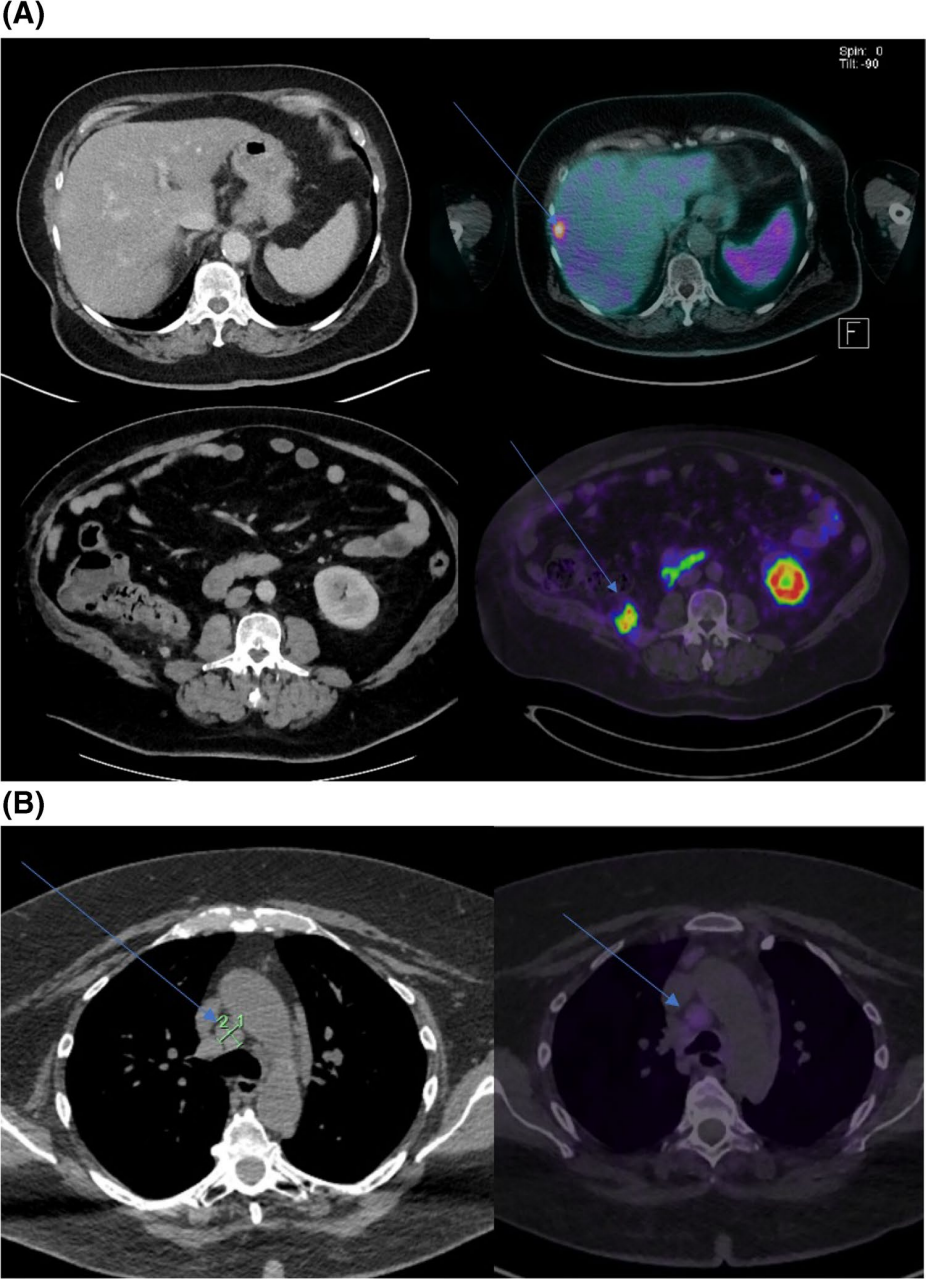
Comparison of PSMA PET compared to CT. A shows better delineation of metastatic disease than CT, B refuted suspected metastatic disease on CT
